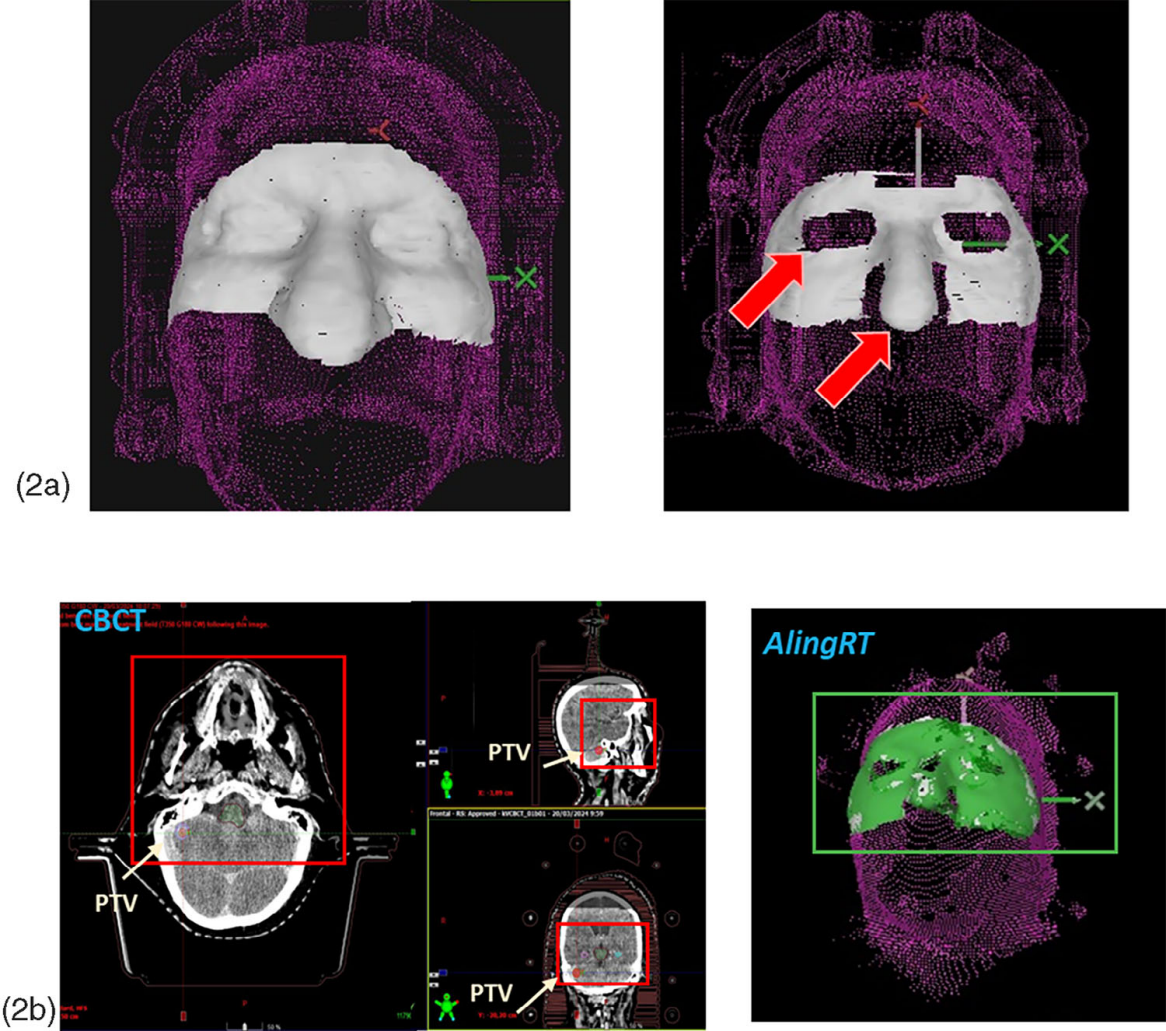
ROI used for SGRT and CBCT. (a) The contoured ROI for surface monitoring included the forehead, eyes, nose, and temporal bones. If the patient blinks too much or has very deep breathing, the eyelids and nose flaps are excluded. (b) A ROI centered on the target site and with similar size to the registration area used in AlignRT is employed to register CBCT with the planning CT images.
